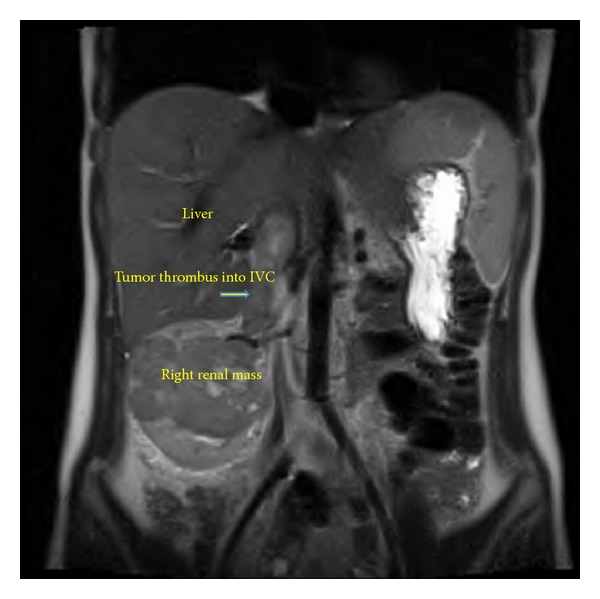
Coronal images of magnetic resonance imaging scan showing tumor thrombus extending into inferior vena cava.
